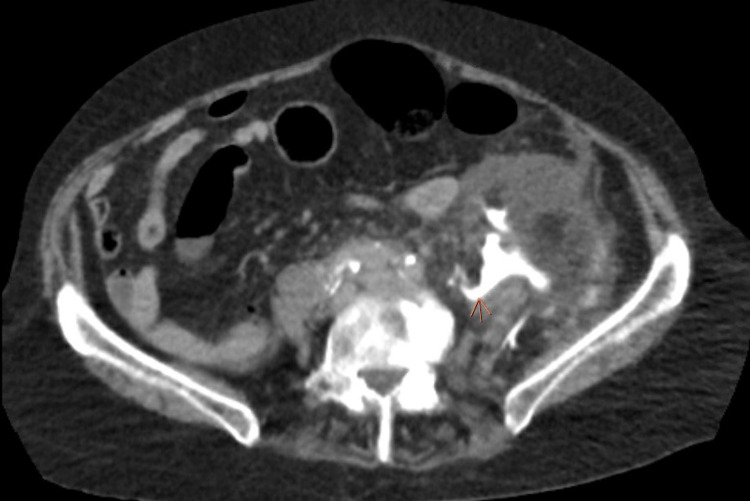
CT abdomen and pelvis showing excreted free contrast seen leaking into pelvis (orange arrow).
